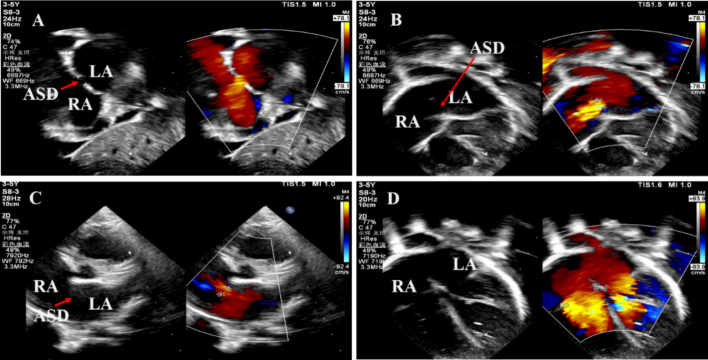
A: A left to right flow can be seen crossed ASD in the subcostal two atrium chamber view by 2D transthoracic echocardiography with color Doppler. B: A left to right flow was shown across ASD in the apical four-chamber view by 2D transthoracic echocardiography with color Doppler. C: A left to right flow was shown across ASD in the parasternal short axis view by 2D transthoracic echocardiography with color Doppler. D: The echocardiography displayed the enlargement of RA and right ventricle. Red arrows indicate the ASD. Notes: LA: left atrium; RA: right atrium; ASD: atrial septal defect. (For interpretation of the references to color in this figure legend, the reader is referred to the web version of this article.)
